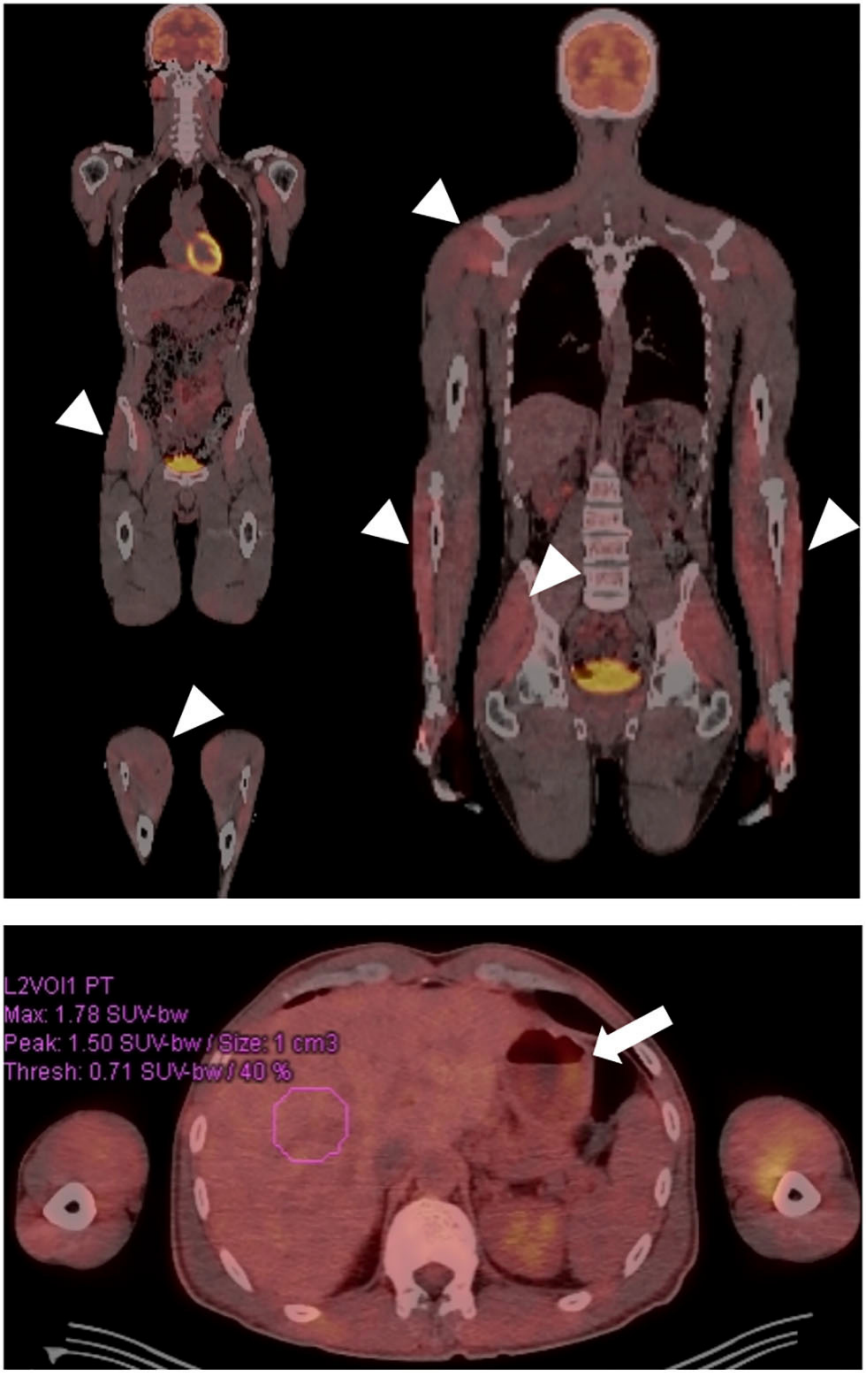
Patient with autoimmune gastroparesis and diffuse myositis. Body FDG-PET revealed diffuse uptake throughout the musculature compatible with the patient's known myositis (arrow heads). Gastroparesis was also incidentally demonstrated, given presence of a large amount of stomach contents despite fasting for >12 h (arrow).
